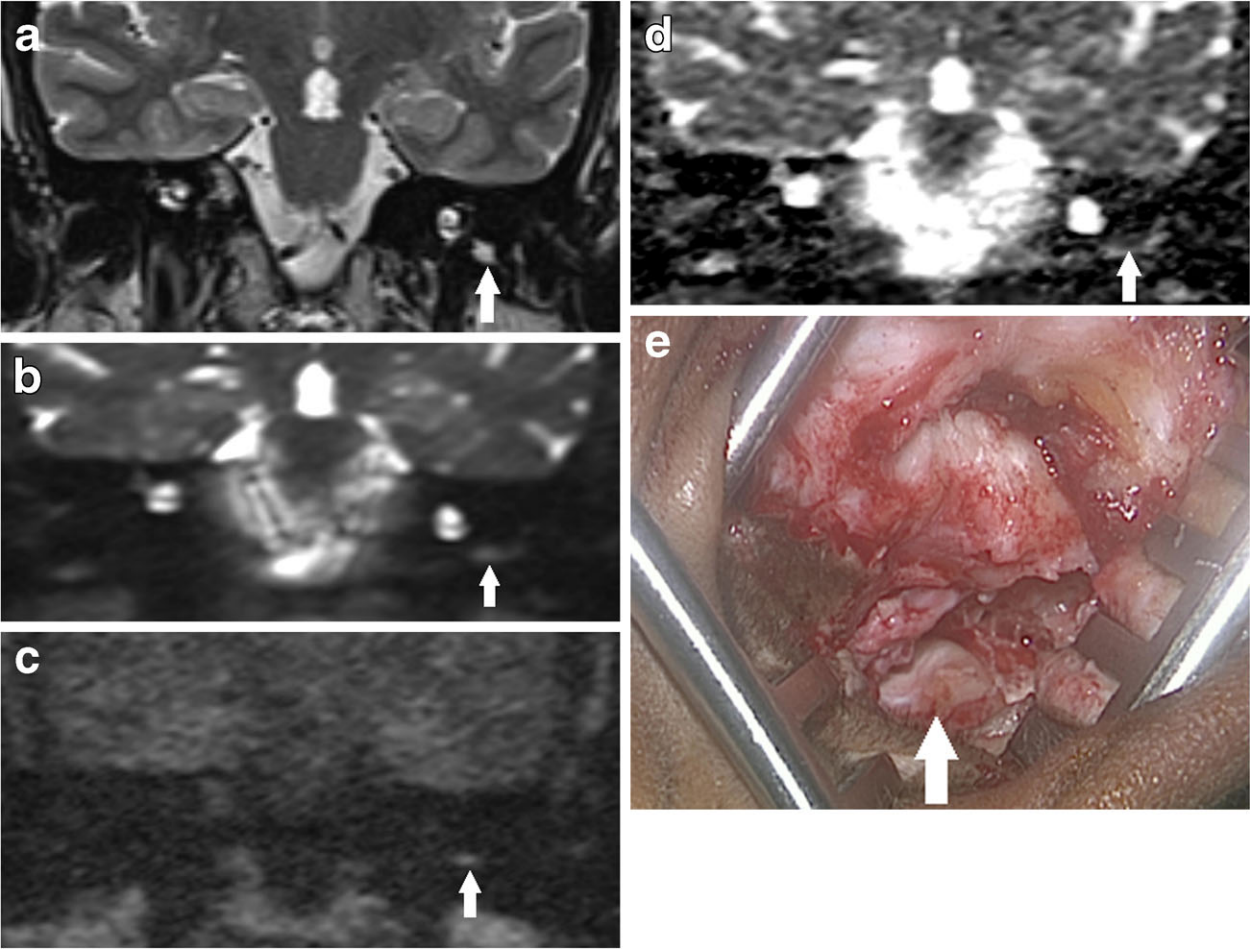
Indeterminate appearance of residual cholesteatoma: Value of ADC. The coronal a T2 weighted and b b0 DWI images show a small focus of high signal soft tissue at the left hypotympanum, which returns just mildly high signal relative to brain tissue on c the b1000 DWI image, raising the possibility of a residual pearl (similar to Fig. 9a). d The ADC map demonstrates low signal and value compatible with cholesteatoma, thereby increasing observer confidence. e This was later confirmed as a 3–4 mm cholesteatoma at the left hypotympanum on second-look surgery
